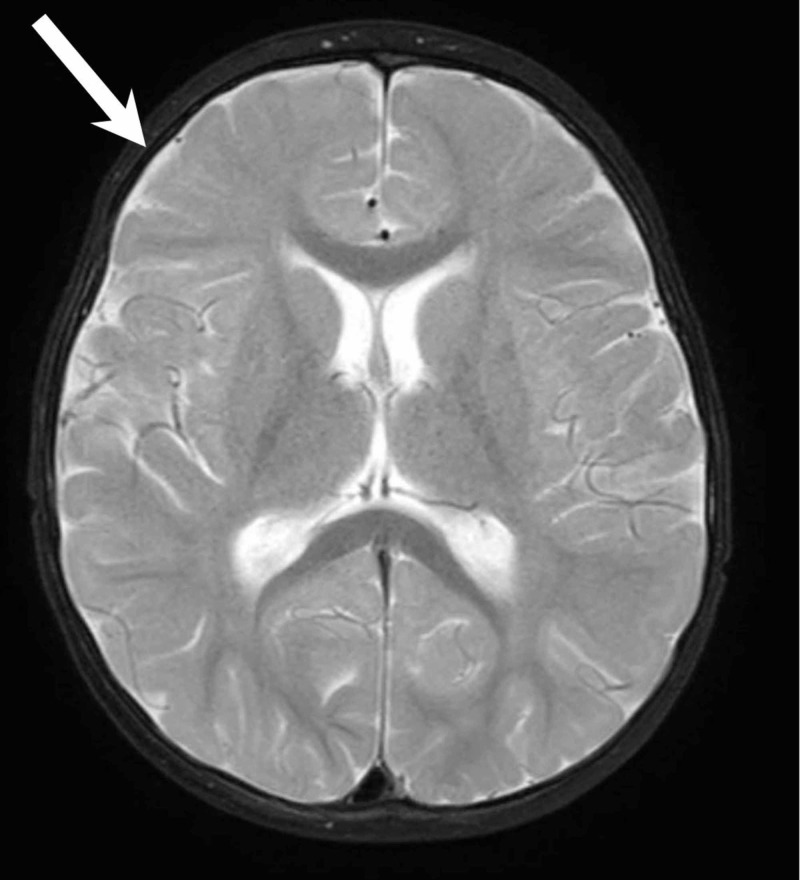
Axial, T2-weighted MRI showing resolution of bilateral subdural empyemas (arrow) as compared to the previous figure.
